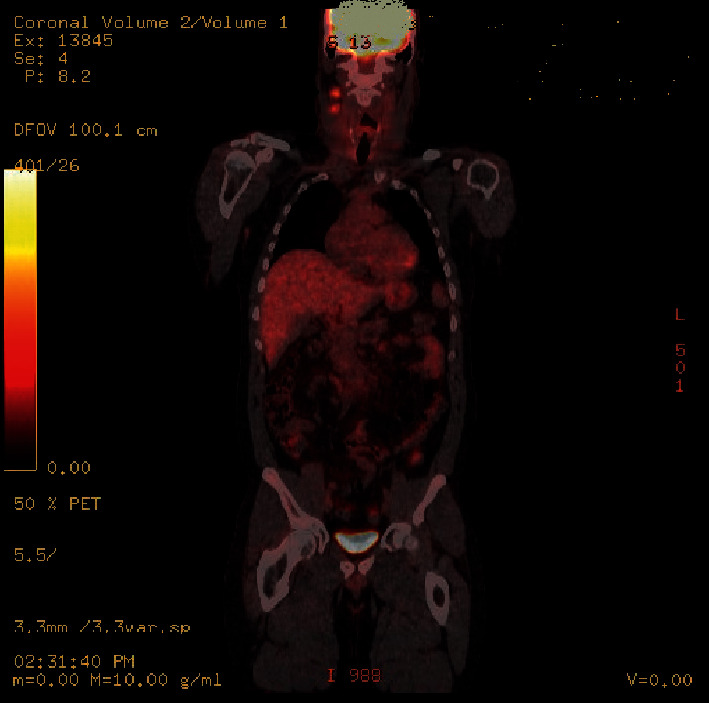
FDG-PET scan showing increase in uptake in two upper cervical lymph nodes.
